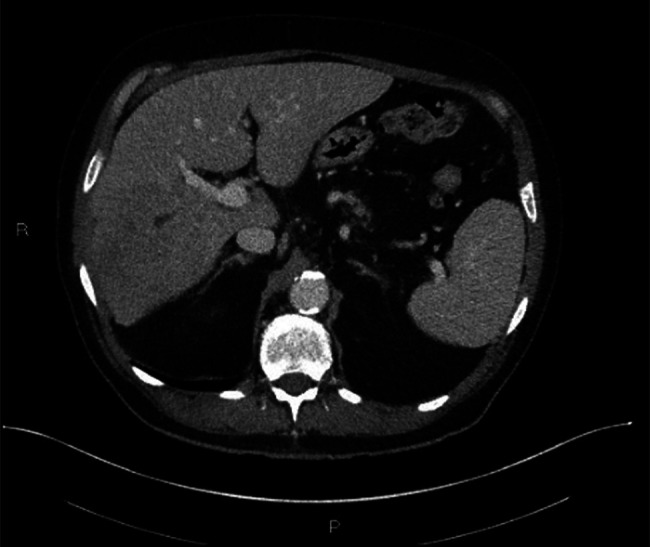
Venous phase computed tomography demonstrated right posterior portal vein thrombosis; raising suspicion for a tumor in vein.
